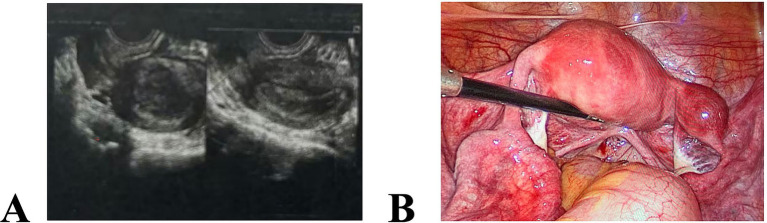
Preoperative pelvic ultrasound images and intraoperative findings of rudimentary horn pregnancy. (A) Preoperatively, the uterus was of normal size, and the endometrium was ~1.5 cm thick; a strip-like anechoic area approximately 0.2 cm in width was observed at the lower end of the uterine cavity. In the right adnexal region, medial to the right ovary, a predominantly solid mixed mass measuring ~2.3 × 2.0 cm was noted, with a small amount of cystic echoes; (B) Intraoperatively, a uterine body located slightly to the left in the pelvis was of normal size but abnormal in shape; on the right, a muscular mass approximately 3.5 cm in diameter was observed, with abundant surface blood supply and a violaceous-red color, and the two were connected by a muscular cord-like tissue.
